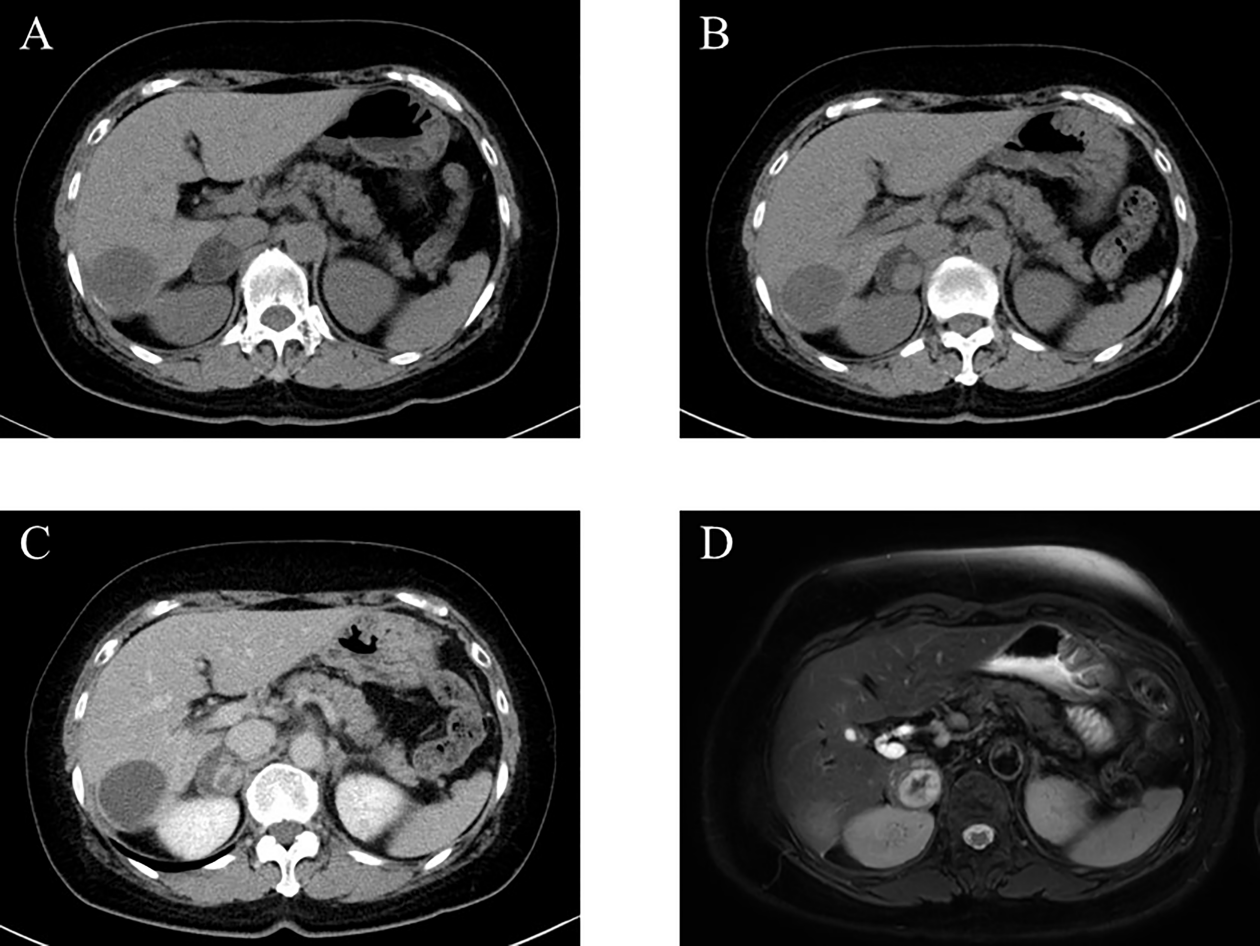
Imaging analysis. (A) Non-enhanced computed tomography (CT) at the first admission. (B, C) Non-enhanced (B) and enhanced (C) CT 3 years after the first admission. (D) T2-weighted magnetic resonance imaging (MRI) at the second admission.
